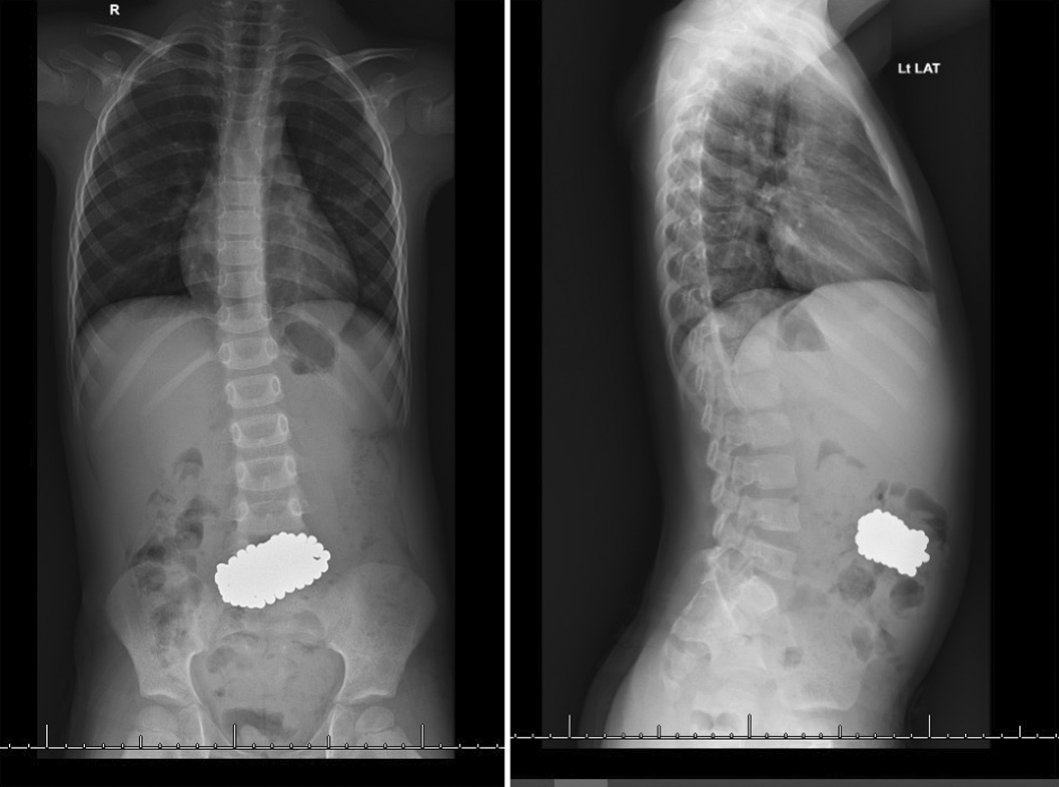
Anteroposterior and lateral radiographs of the abdomen demonstrating a large conglomeration of magnetic beads, appearing as a radio‐opaque cluster, localized in the distal gastrointestinal tract.
